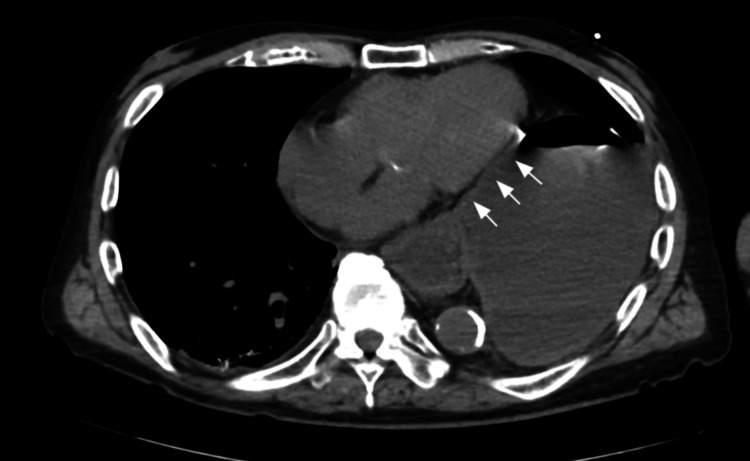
Axial CT at the time of hemodynamic collapse. The distended stomach compresses the heart, as indicated by the arrows.
